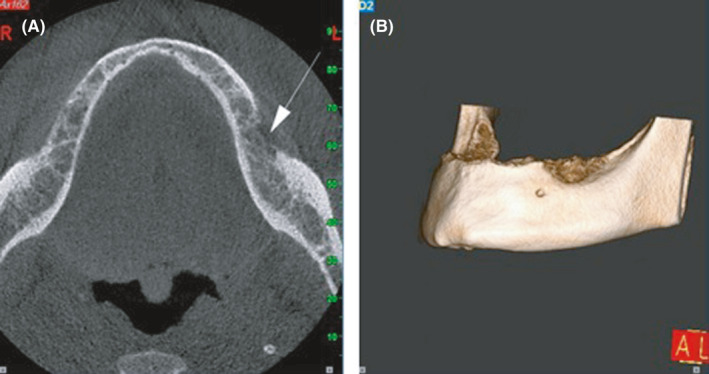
(A) Axial view of cone beam computed tomography (CBCT) shows an ill‐defined mass with buccal cortical plate invasion. (B) Three‐dimensional CBCT demonstrates left‐sided osteolytic destruction of the mandible
